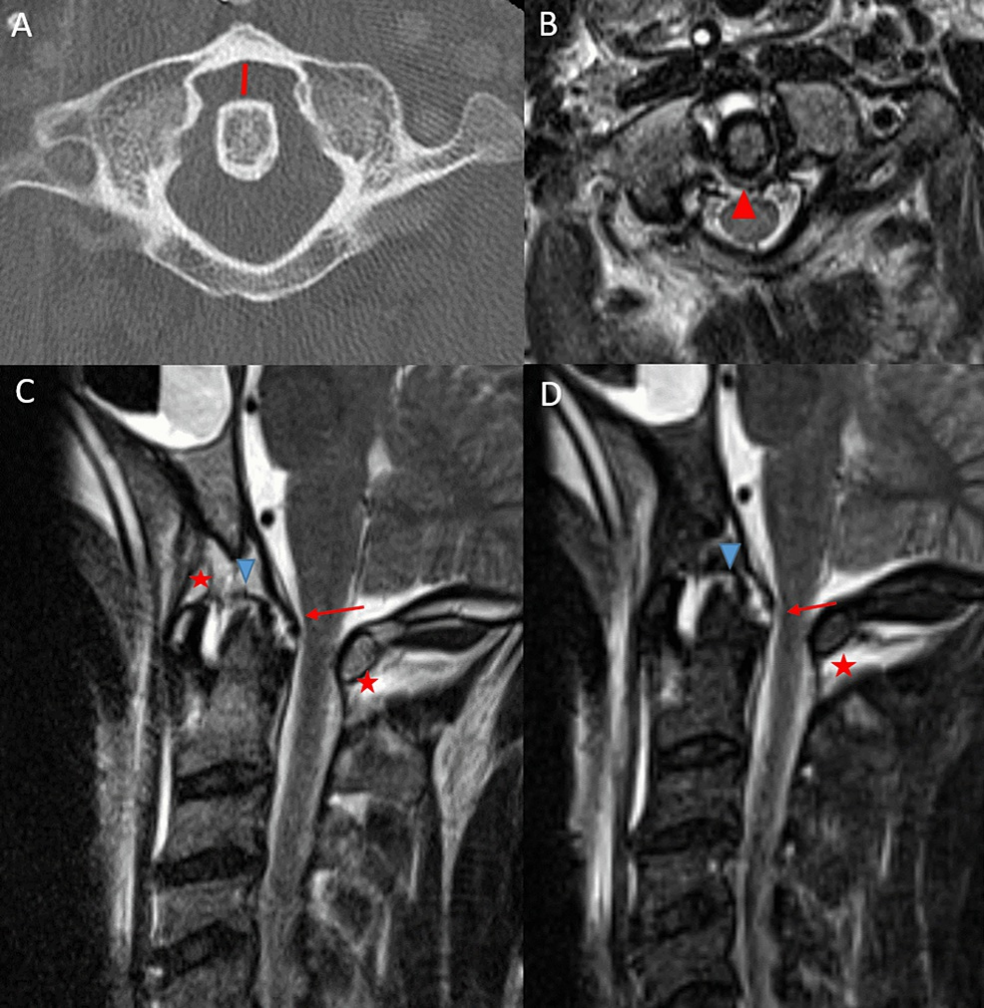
Axial CT of the cervical spine (A) in a 48-year-old trauma patient status post MVA demonstrating widening of the anterior atlanto-dens interval (red line) without a C1 fracture. Axial T2-weighted MRI (B) in the same patient demonstrating a central transverse atlantal ligament tear (red arrow). Sagittal T2-weighted (C) and STIR-weighted (D) MRI of the cervical spine in the same patient demonstrating subclival tectorial membrane tear (red arrows), right alar ligament tear (blue arrowheads), and anterior and posterior atlanto-occipital membrane complex tears (red stars). The majority of observed patients in our study with atlantoaxial instability did not have major craniocervical junction ligament tears. CT: computed tomography; MVA: motor vehicle accident; MRI: magnetic resonance imaging; STIR: short tau inversion recovery
